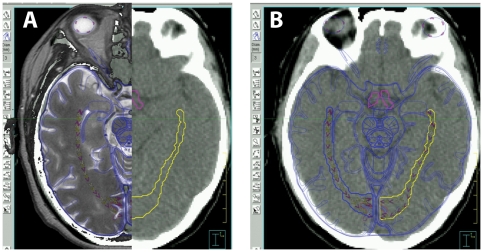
Anatom-E digital atlas. For structure delineation, the display of treatment planning system (Pinnacle) is mirrored to a second computer monitor, and the Anatom-E images are superimposed (A) and adjusted and scaled to achieve registration with the planning CT images. Anatom-E software controls allow blending (B) of the MRI and wireframe views for ease of registration and structure identification.
